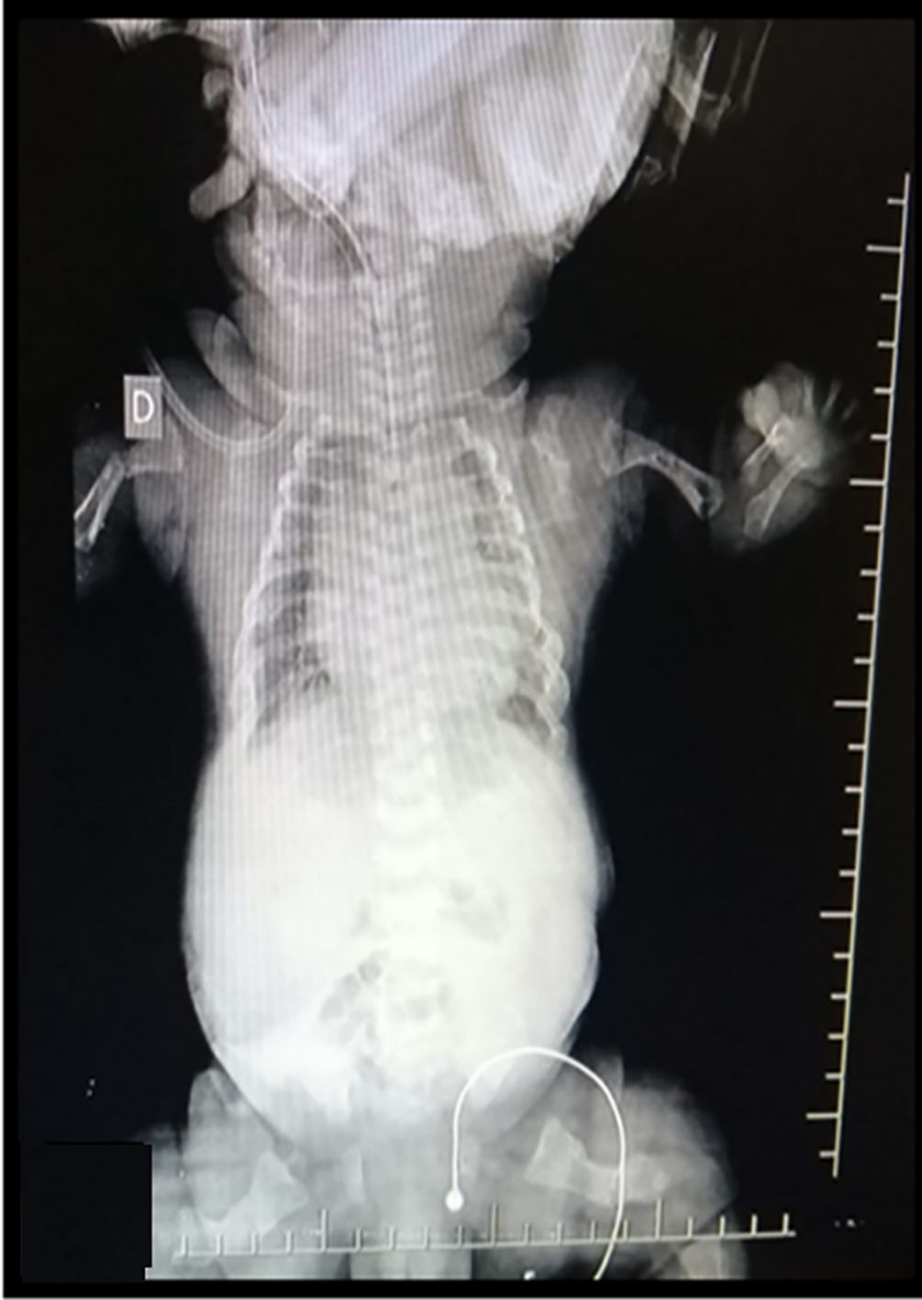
Radiographs from proband which presented hypomineralization and lack of growth of the long bones.
